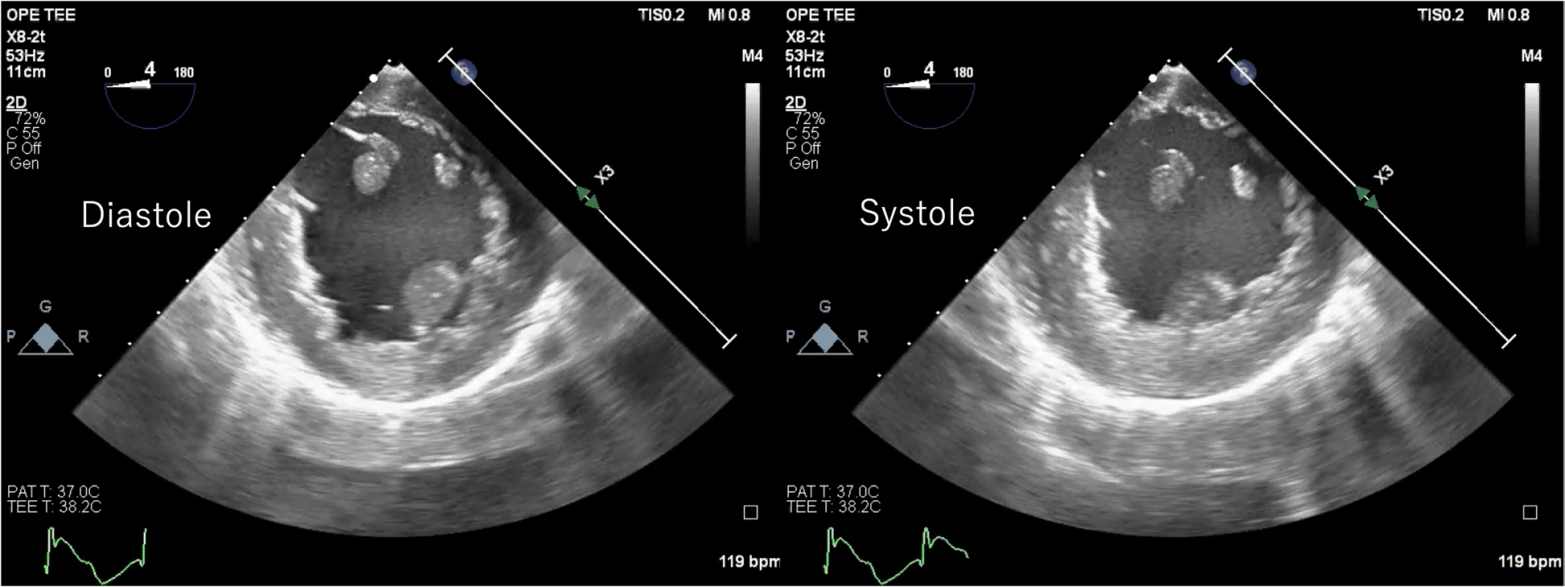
Regional wall motion abnormality observed on a mid-esophageal short-axis view. Transesophageal echocardiographic images obtained during diastole (left) and systole (right) show a regional wall motion abnormality extending from the lateral to the inferior wall
